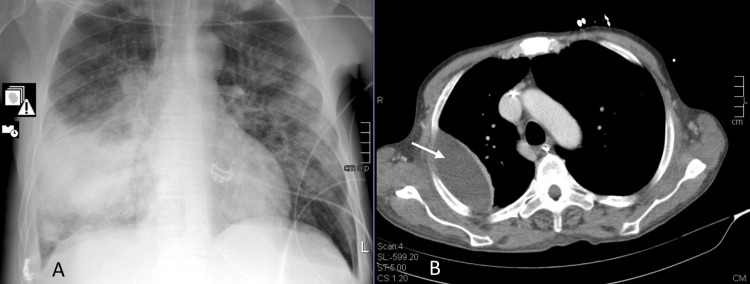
X-ray and CT scan of the patient in case 1 A: X-ray at admission – right middle and lower consolidation. B: CT scan made on day 15 of hospital stay showing one of the lung abscesses later drained.
